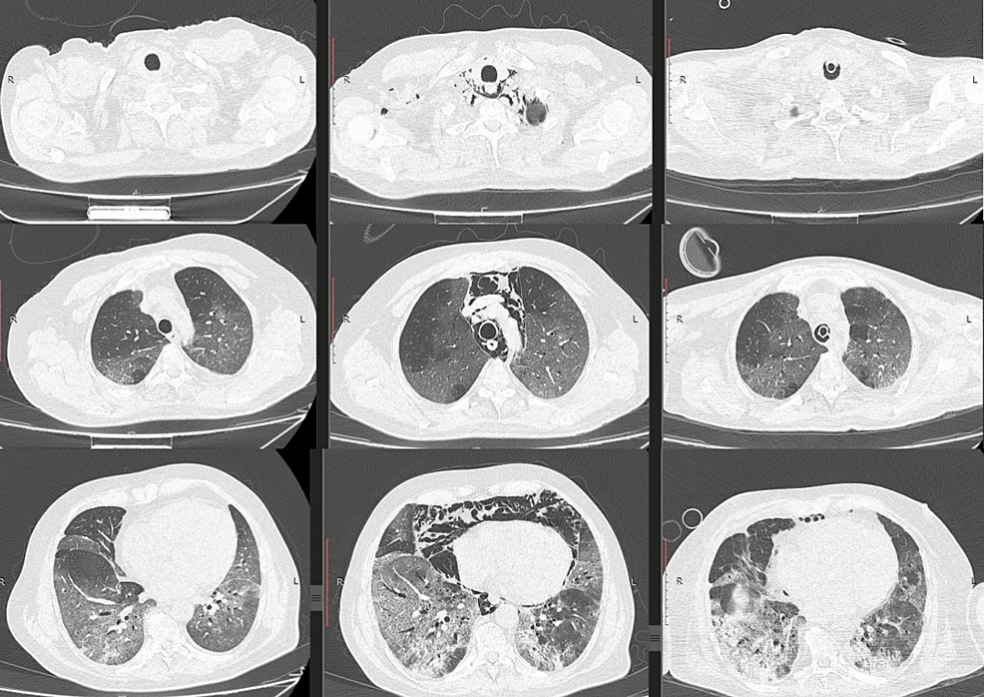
Series of images of computed tomography scan of a 51-year-old male with severe pneumonia done on days three, 10, and 15 after admission. The presence of spontaneous pneumomediastinum and subcutaneous emphysema on day 10 is notable, with the evident regression of the lesions on day 15, even when intubation and mechanical ventilation were needed.
